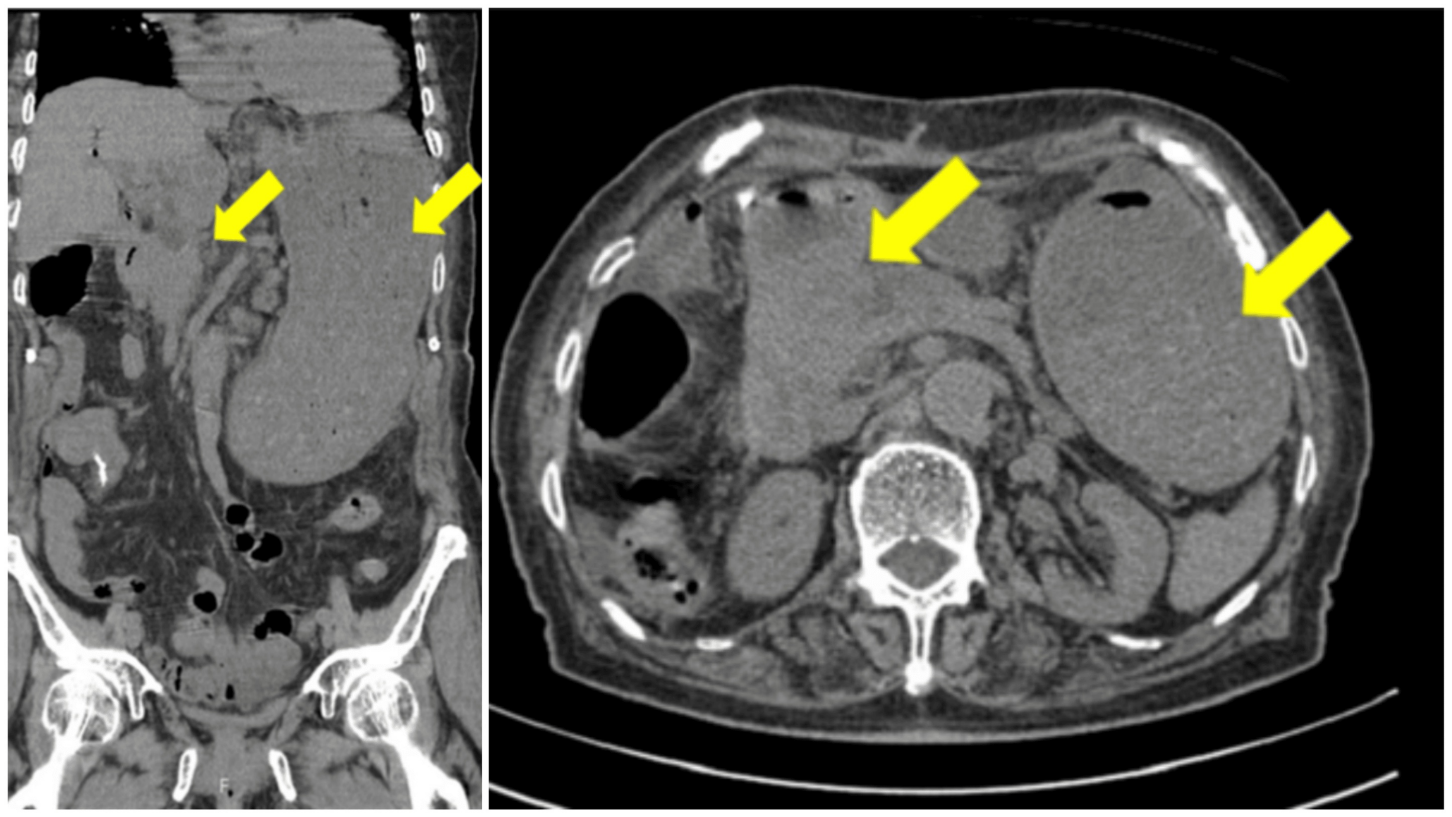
CT images of pancreatic head cancer at admission CT revealed tumor growth of the pancreatic head with duodenal invasion. Gastric distension due to duodenal obstruction was also observed.
